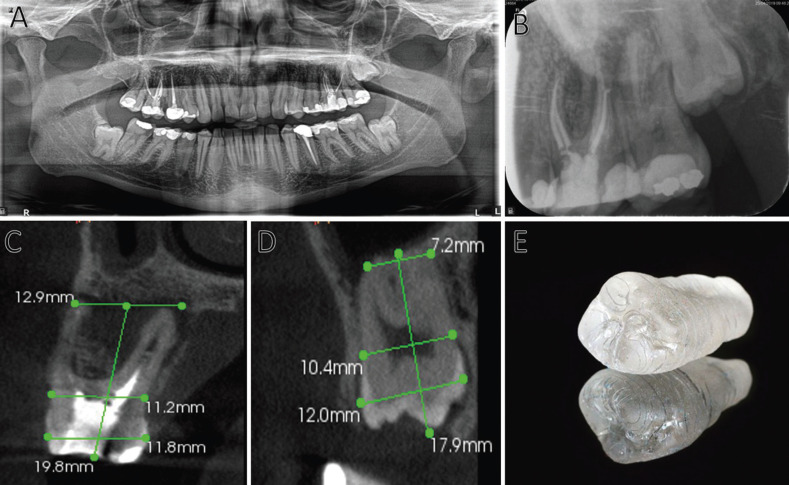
A. Orthopantomography of the initial study. B. Initial diagnostic periapical radiography. C. Parasagittal section of the CBCT of tooth 2.6. Measurements reflecting the dimensions of the tooth. D. Parasagittal section of tooth 2.8. Measurements reflecting the dimensions of the tooth. E. 3D- printed replica of 2.8.
